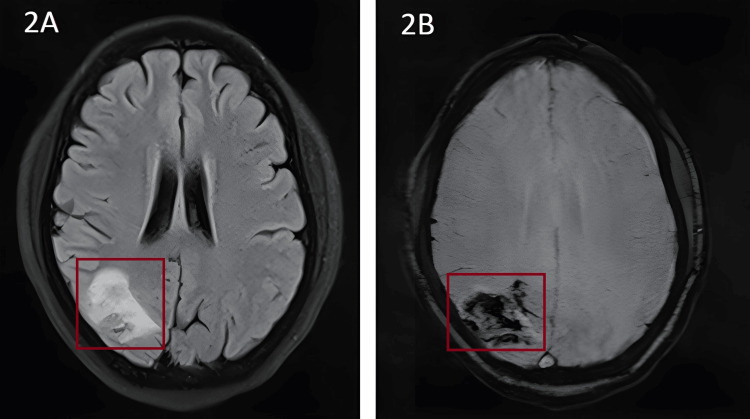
MRI brain findings 2A: MRI brain T2 FLAIR showing heterogeneously hyperintense area involving cortical and subcortical regions of the right parieto-occipital region. 2B: MRI brain SWI showing blooming suggestive of hemorrhagic venous infarct in the same area FLAIR: fluid-attenuated inversion recovery; MRI: magnetic resonance imaging; SWI: susceptibility-weighted imaging
